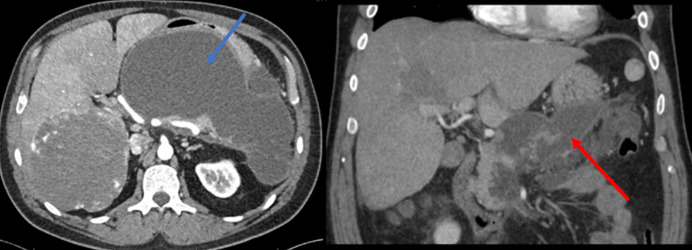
Axial and coronal postcontrast computed tomography scan. Thirty-three days after embolization. Multiple pancreatic pseudocysts. The largest pseudocyst now measures 22.3 ×10.5 × 10 cm (blue arrow) and development multiloculated peripancreatic fluid collections/walled off necrosis (red arrow).
